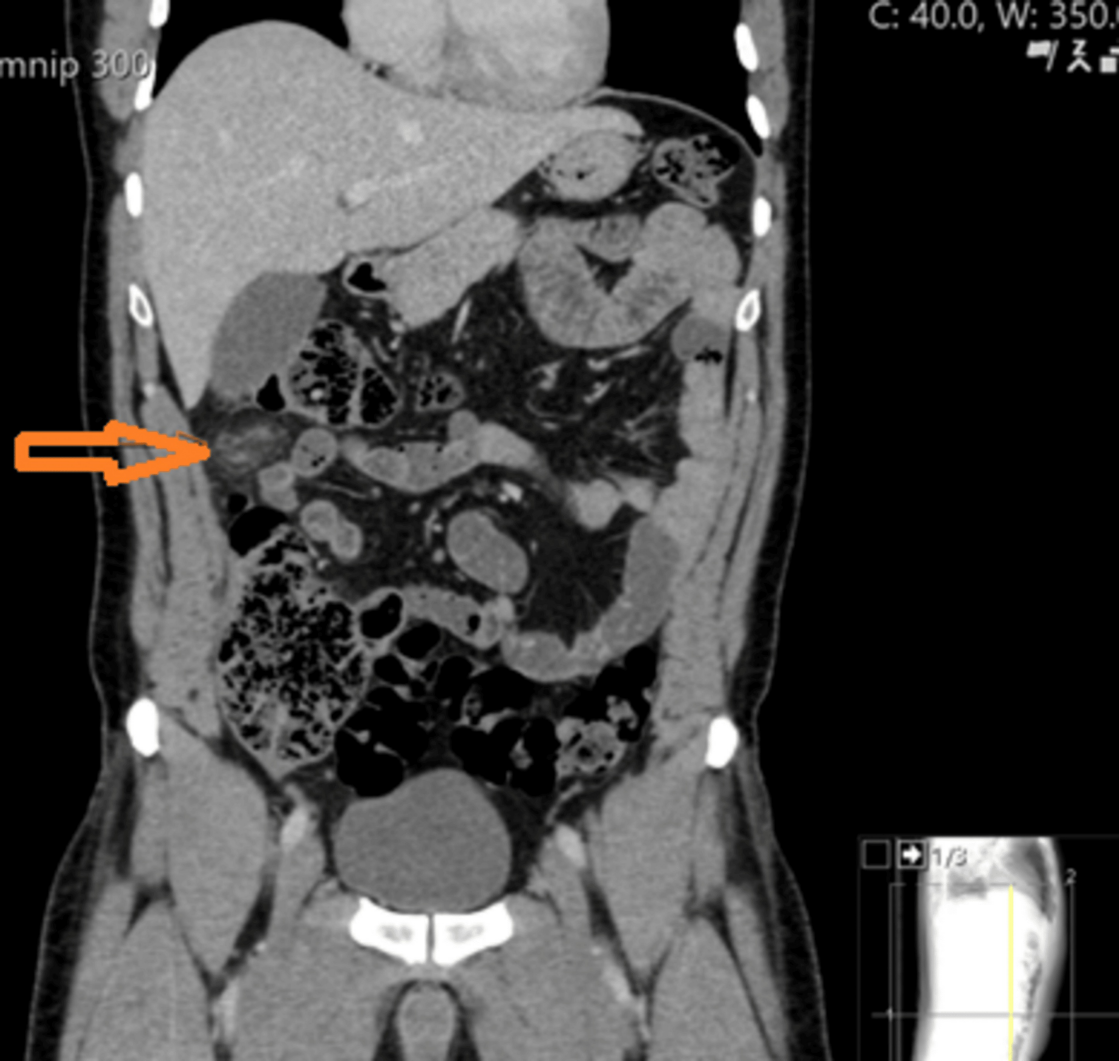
CT coronal view showing features of fat density ovoid structure with mild inflammatory fat stranding with some adjacent peritoneal thickening A 32-year-old male patient presented to our hospital with right upper abdominal pain (no patient identifiers on the CT film).
